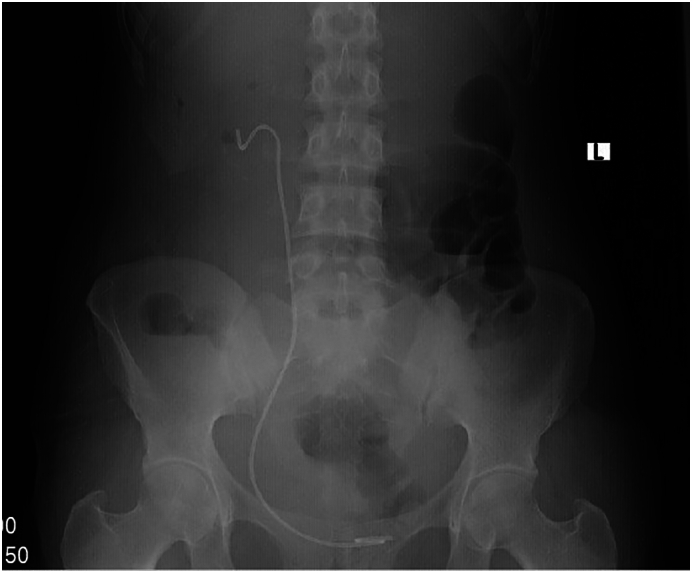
Post-operative control X-ray; it shows a well-placed stent with no residual fragment.
